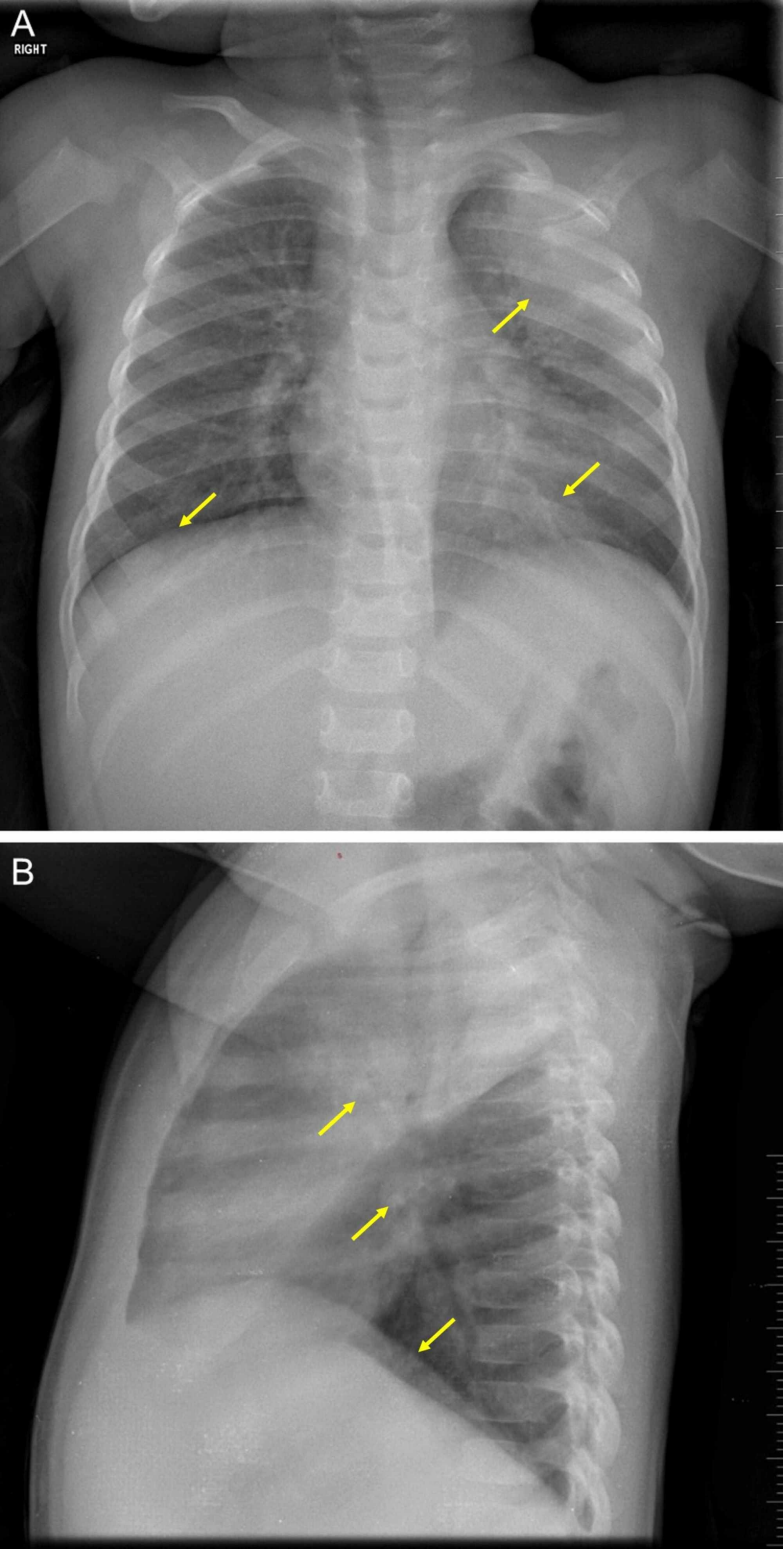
A chest radiograph. Imaging of the infant’s chest shows large consolidation at the left apical lobe with bronchial infiltrates that are dominant at the left base, and asymmetrical lung bases (A). The heart size is normal, the rib cage is intact, and the diaphragmatic arches are in normal position (A). A discrete blunting at the left pleural sinus can be observed (B).
